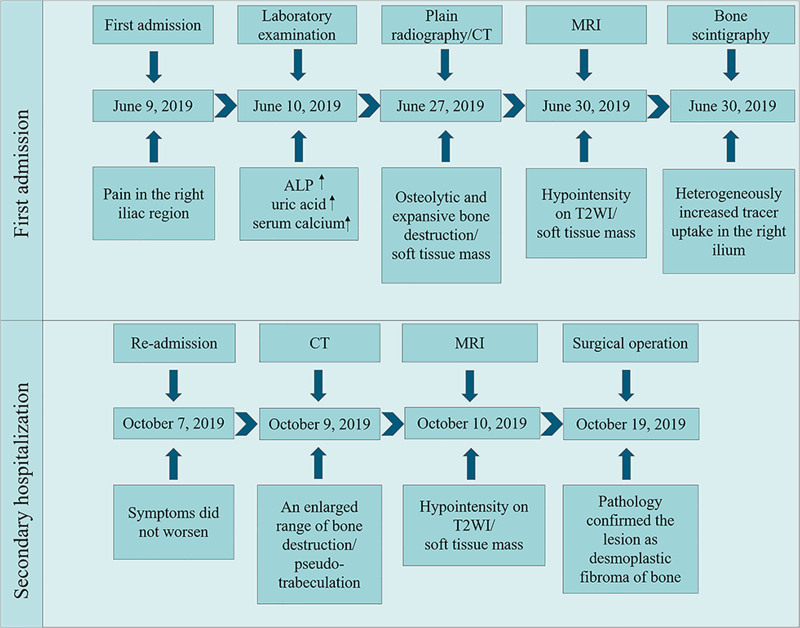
The timeline of the radiological imaging examination and diagnostic process of the patient in the present case. ALP = alkaline phosphatase, CT = computed tomography, MRI = magnetic resonance imaging.
